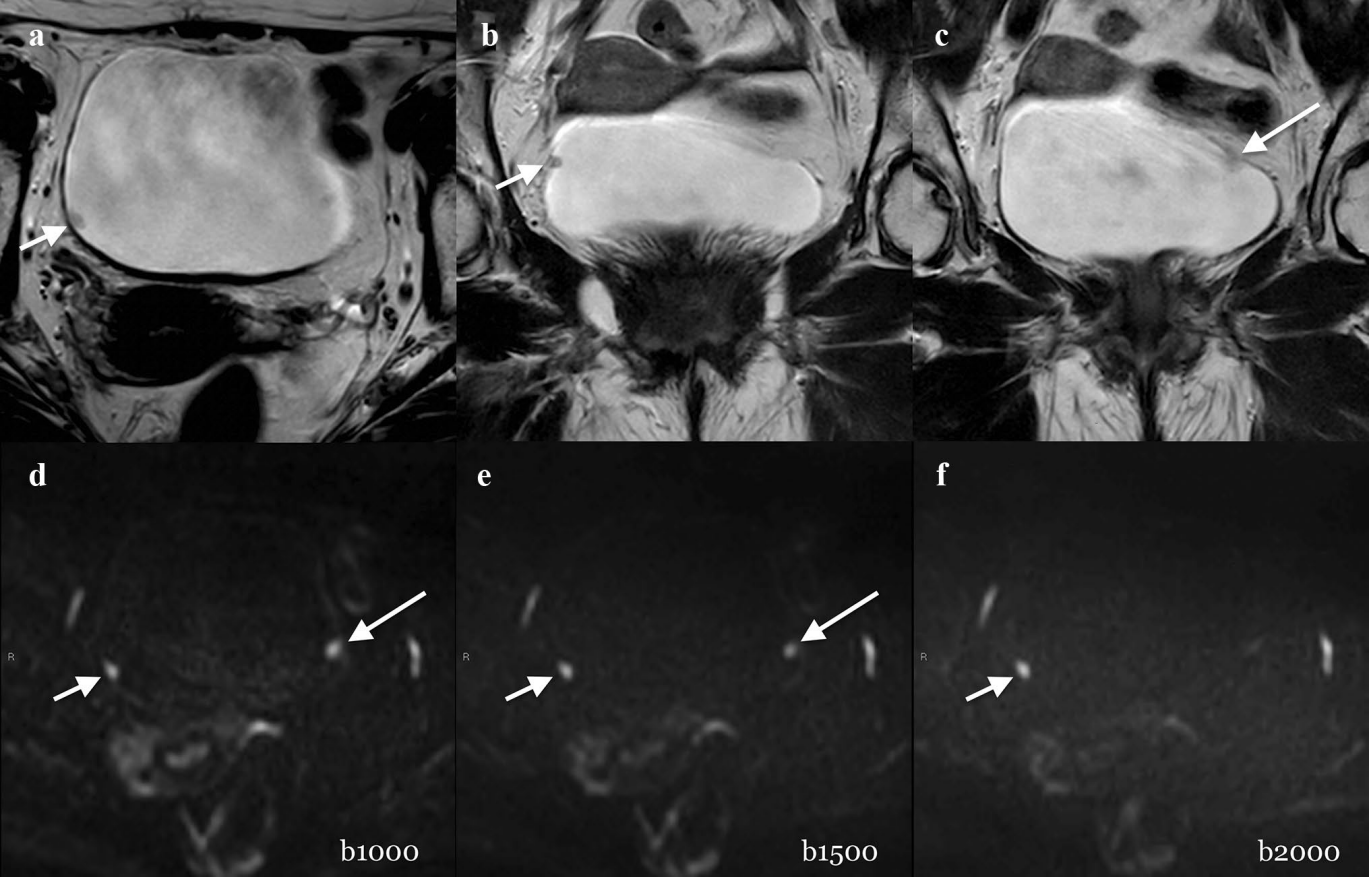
Example of a not muscle-invasive BC classified incorrectly. A 73-year-old man with hematuria and two polyps, documented after flexible cystoscopy, underwent MRI before primary TURB. Axial (a) and coronal (b, c) T2W imaging (T2) showed a small (4 mm) polypoid lesion on the right wall of the bladder (short arrow in a and b). The lesion was well detected by the three readers regardless the image set and was scored as VI-RADS 1 (short arrow in d, e, and f). Another slightly visible small (4 mm) non-muscular invasive lesion (VI-RADS 1) on the left wall of the bladder was suspected on T2 images (long arrow in c). However, it was definitely detected by the three readers only when reading b1000 and b1500 images (long arrow in d and e), but not on b2000 images. T stage after TURB was LG-T1 (TURB). After four weeks, Re-TURB was performed and it confirmed the absence of residual tumor. DWI = diffusion-weighted imaging; HG = high grade; MRI = magnetic resonance imaging; T2W = T2 weighted; TURB = transurethral resection of the bladder; VI-RADS = Vesical Imaging-Reporting and Data System
